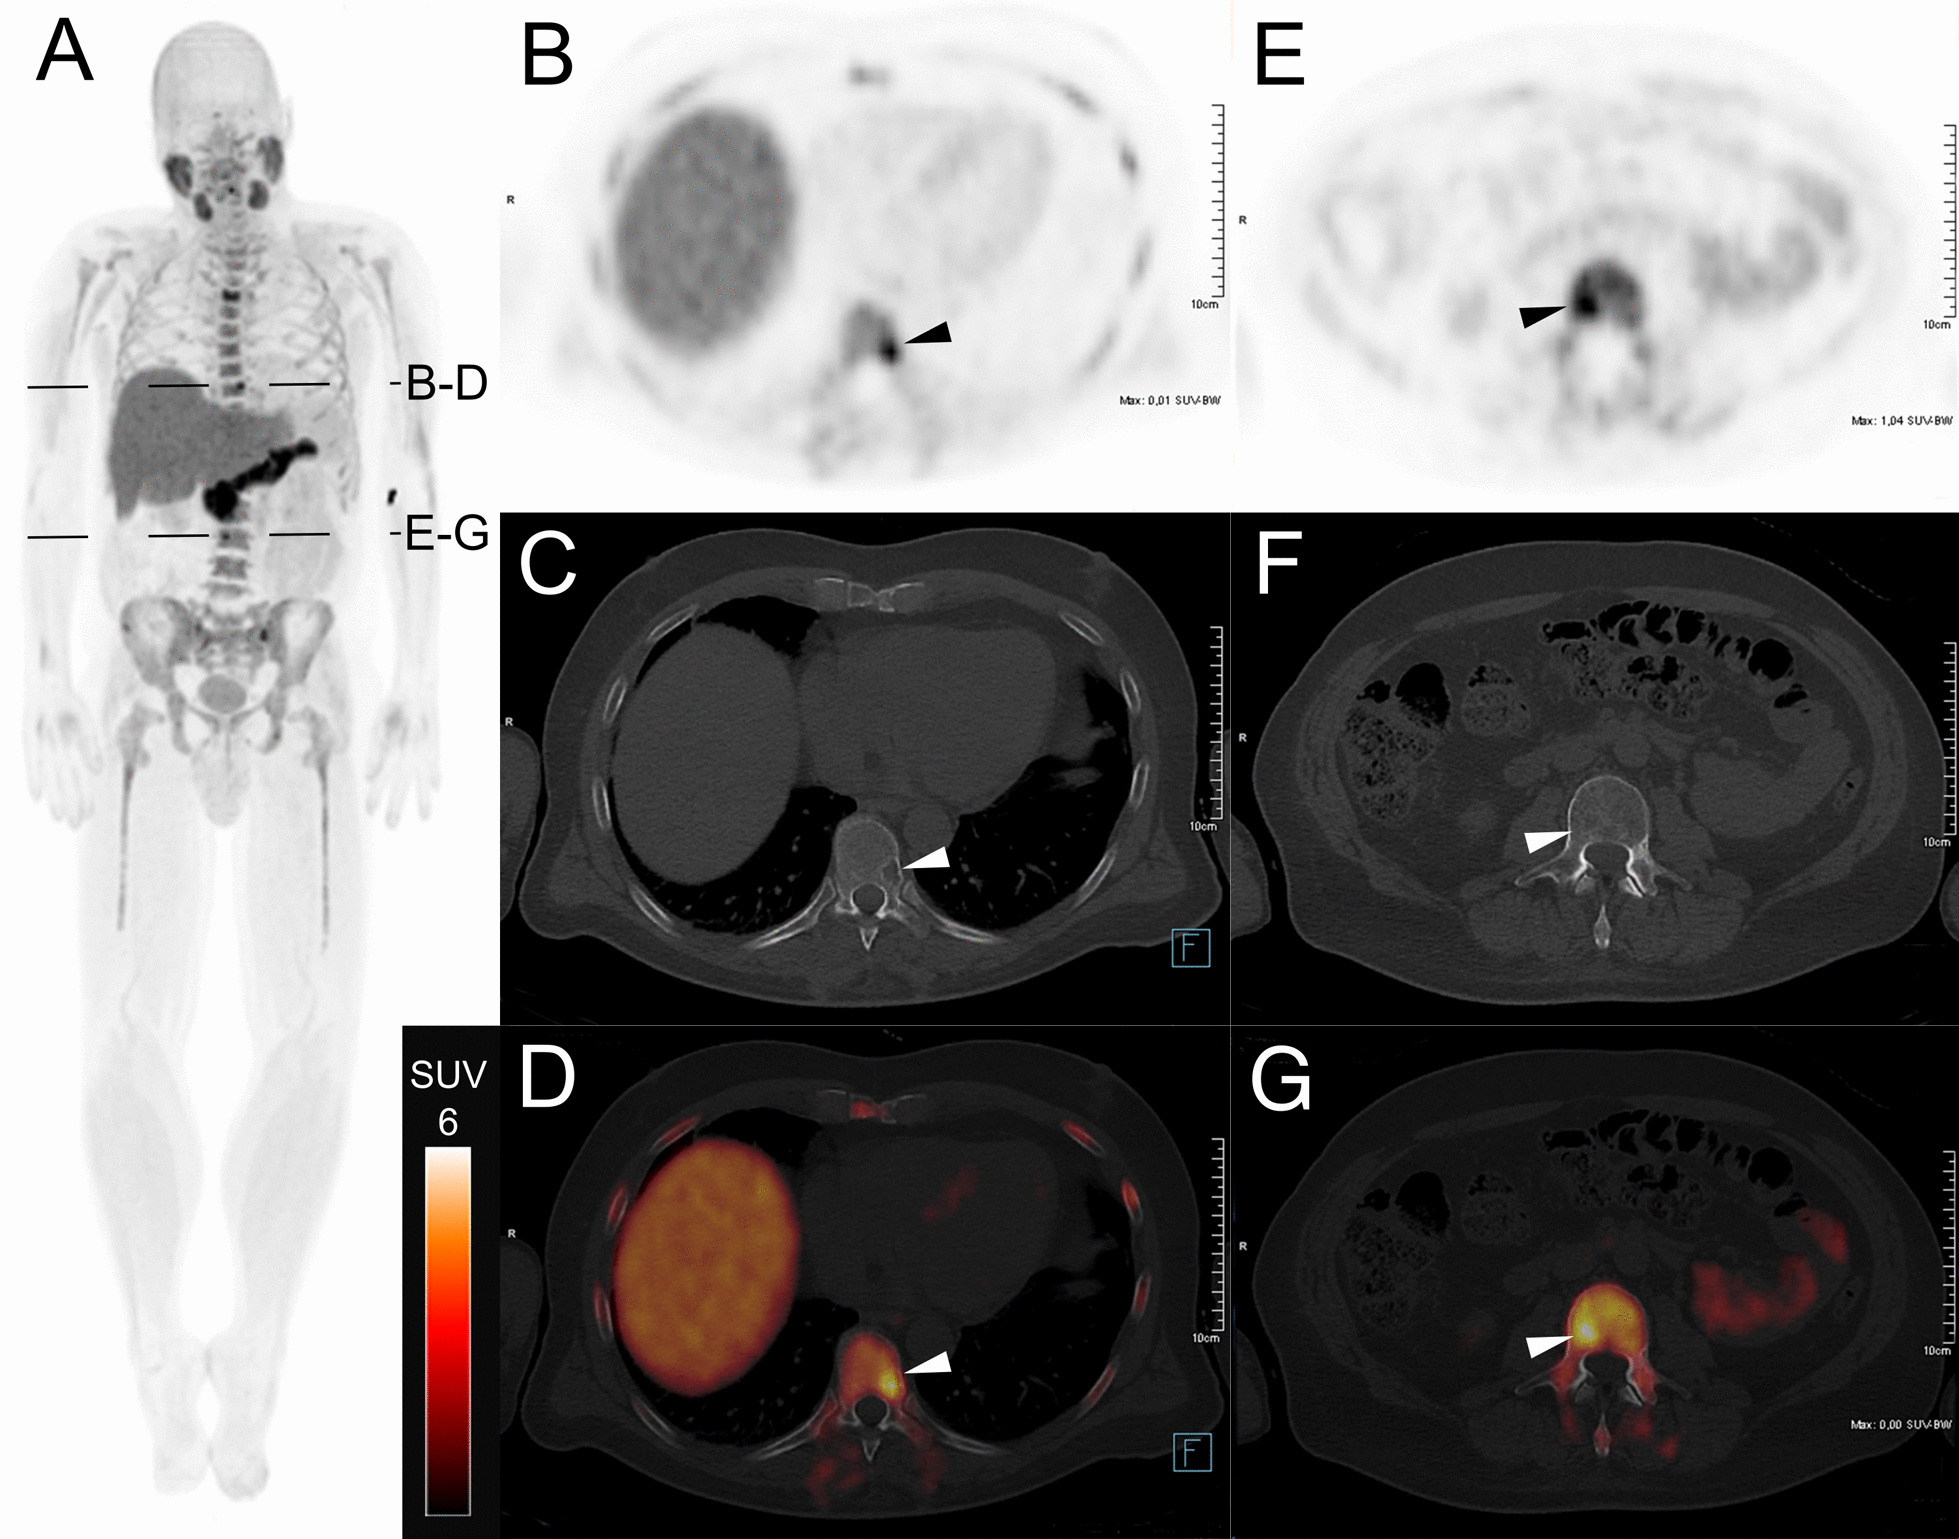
[^18^F]-FACBC PET/CT-scan of a MM patient. A Full body MIP. Axial sections of this patient’s PET (B/E), CT (C/F) and PET/CT fusion (D/G) are depicted. Position of the axial sections is indicated in the MIP (A). Concordant lesion on PET and CT (B–D; SUVmean 8.0; SUVmax 13.1), where the PET-signal corresponds to osteolysis on CT. [^18^F]-FACBC allowed the localization of discordant lesions in PET that were not distinguishable from bone inhomogeneity in CT (E–F; SUVmean 7.9; SUVmax 9.5)
